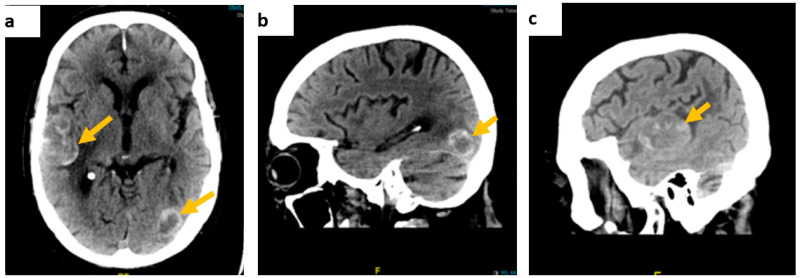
Contrast-enhanced brain CT scan images. (a) On axial view, a right temporoparietal lobe lesion measuring approximately 54 × 33 mm and a smaller left temporal lesion, (b) on sagittal view, a left occipital lobe lesion, measuring approximately 23 × 18 mm, and (c) the right temporal lesion on the sagittal axis.
